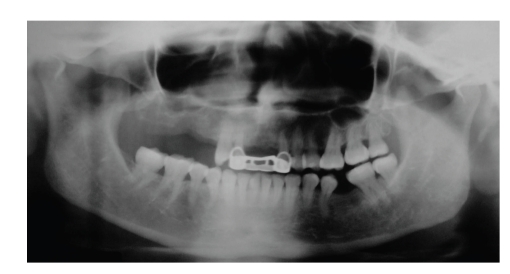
Panoramic X-ray shows a complete postoperative view without the foreign body.
